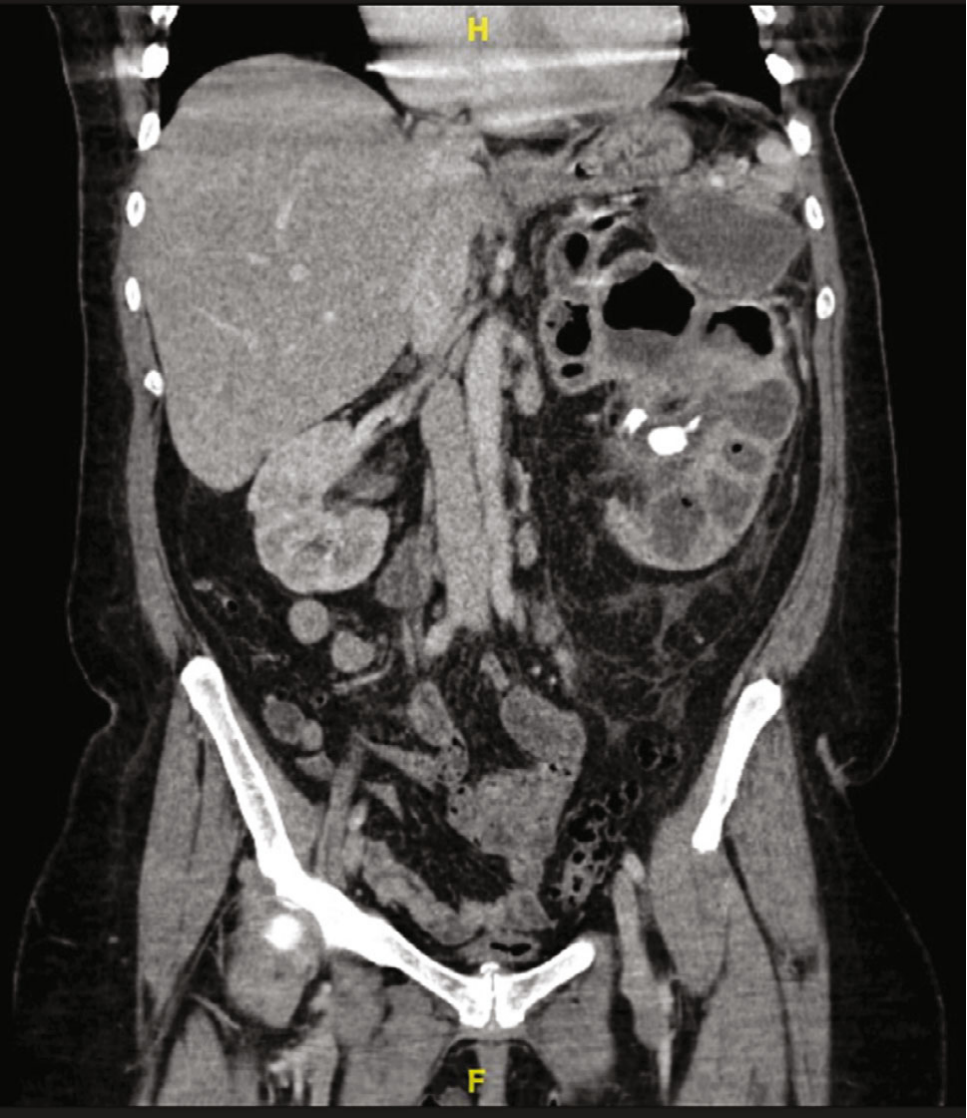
CT abdomen and pelvis with contrast coronal view depicts the characteristic “bear paw” sign seen in xanthogranulomatous pyelonephritis.
